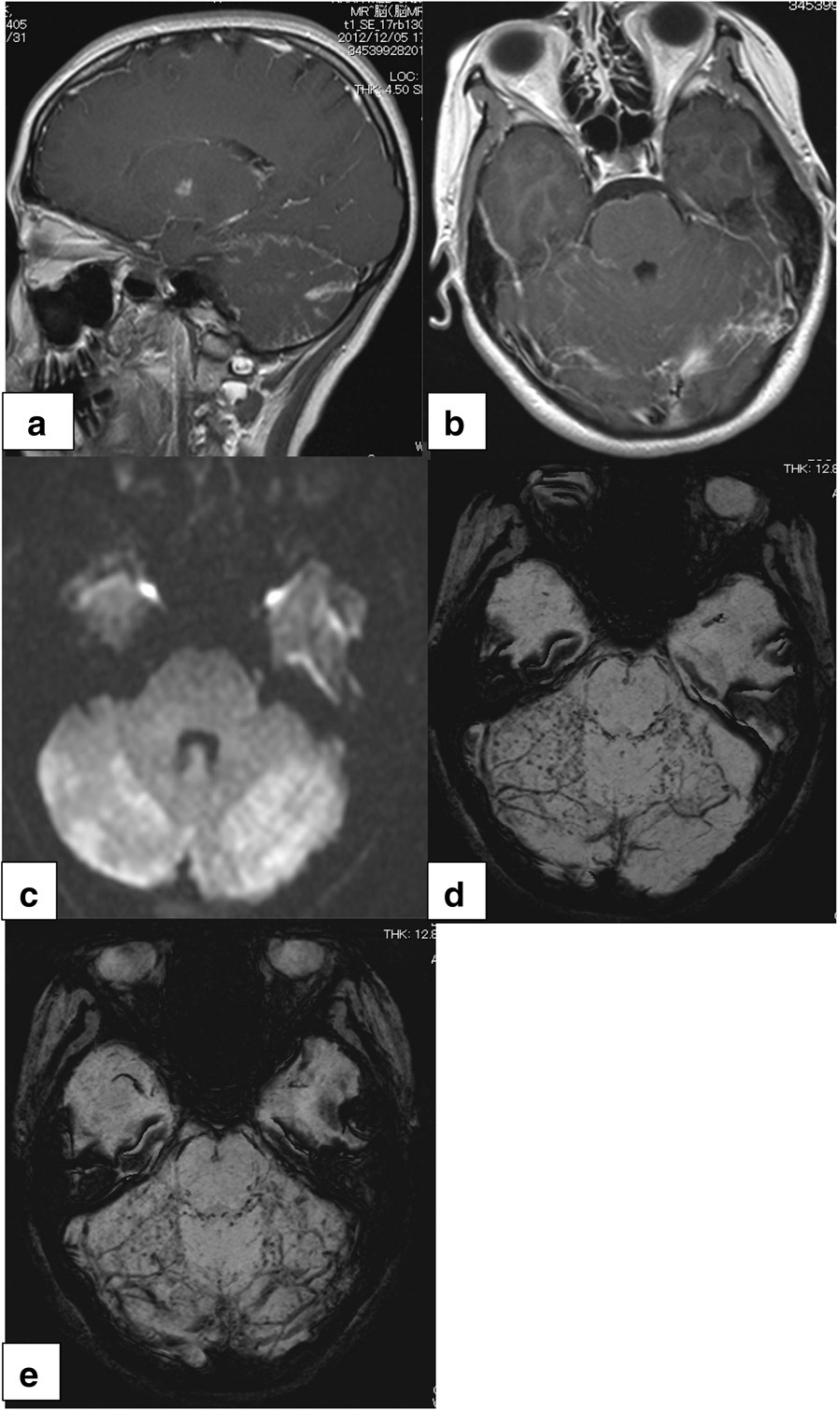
Brain magnetic resonance imaging of the patient. Postcontrast T1-weighted saggital and axial imaging (a, b) showed enhancement in subarachoid space of cerebellum, typically in the great horizontal fissure. Diffusion-weighted imaging (c) showed high-intensity area in the great horizontal fissure. Susceptibility-weighted imaging (d) showed nonlinear multiple low intensity spots. These multiple spots are not enhanced in axial postcontrast T1-weighted image (b). Follow up MRI on day 42 showed that the multiple low-intensity spots persisted, but were disappearing (e)
